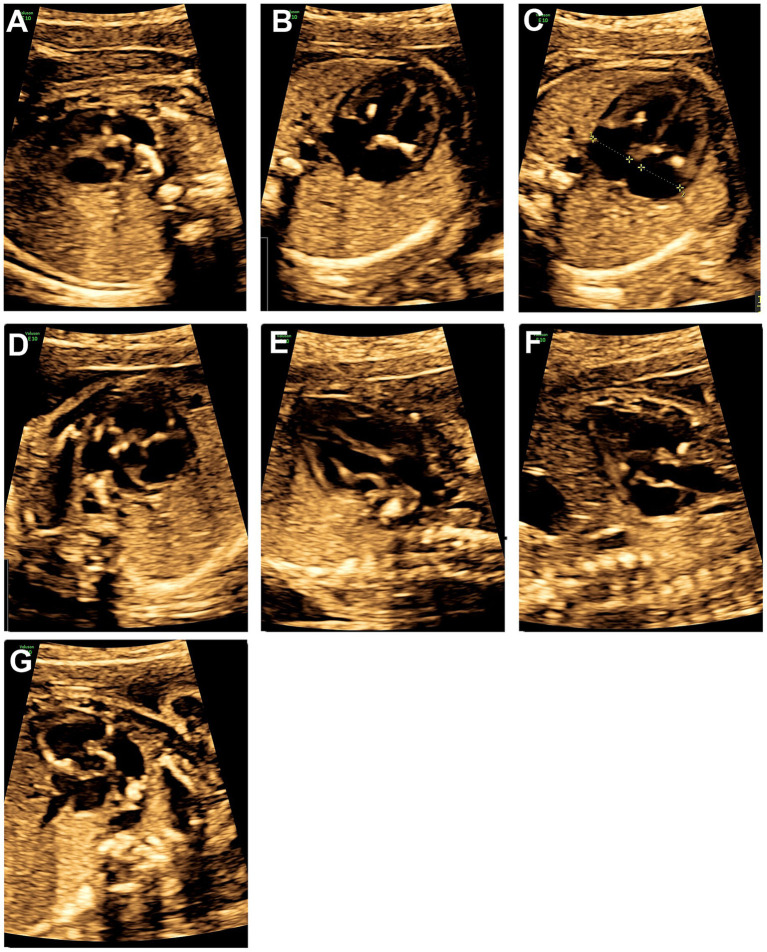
Fetal echocardiogram at 24 weeks. (A) Calcification of pulmonary valve, main pulmonary artery and ductus arteriosus wall, (B) calcification of the tricuspid valve and left ventricular chordae tendineae, (C) calcification of the mitral valve apex, (D) calcification of the septum of the main pulmonary artery trunk, (E) calcification of the aortic origin, (F) calcification of the aortic valve and vessel wall, (G) calcification of the walls of the heart valves and great vessels.
